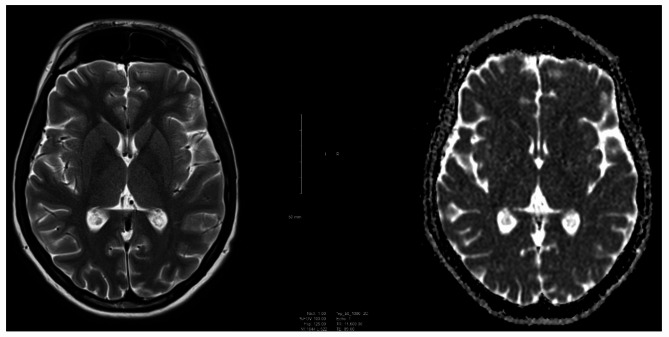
Axial T_2_-weighted (left) vs. apparent diffusion coefficient (ADC) map (right) MRI scans
